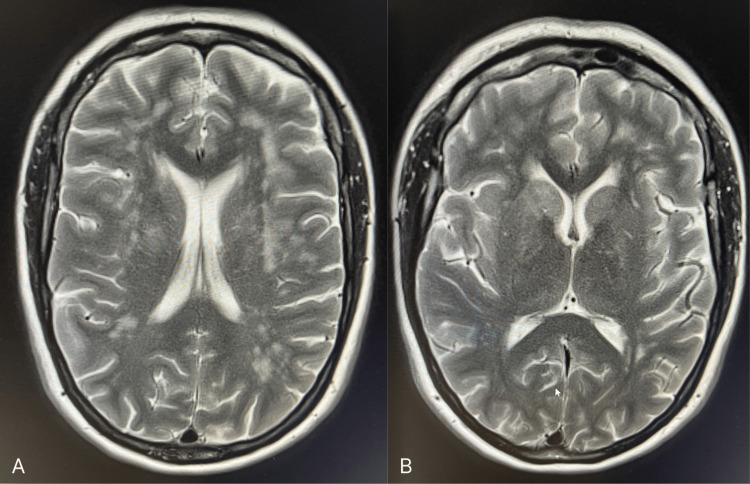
MRI scan of the brain showing white matter changes notable in the frontal lobes (A) as well as a signal dropout in the lentiform and caudate nuclei region (B)
